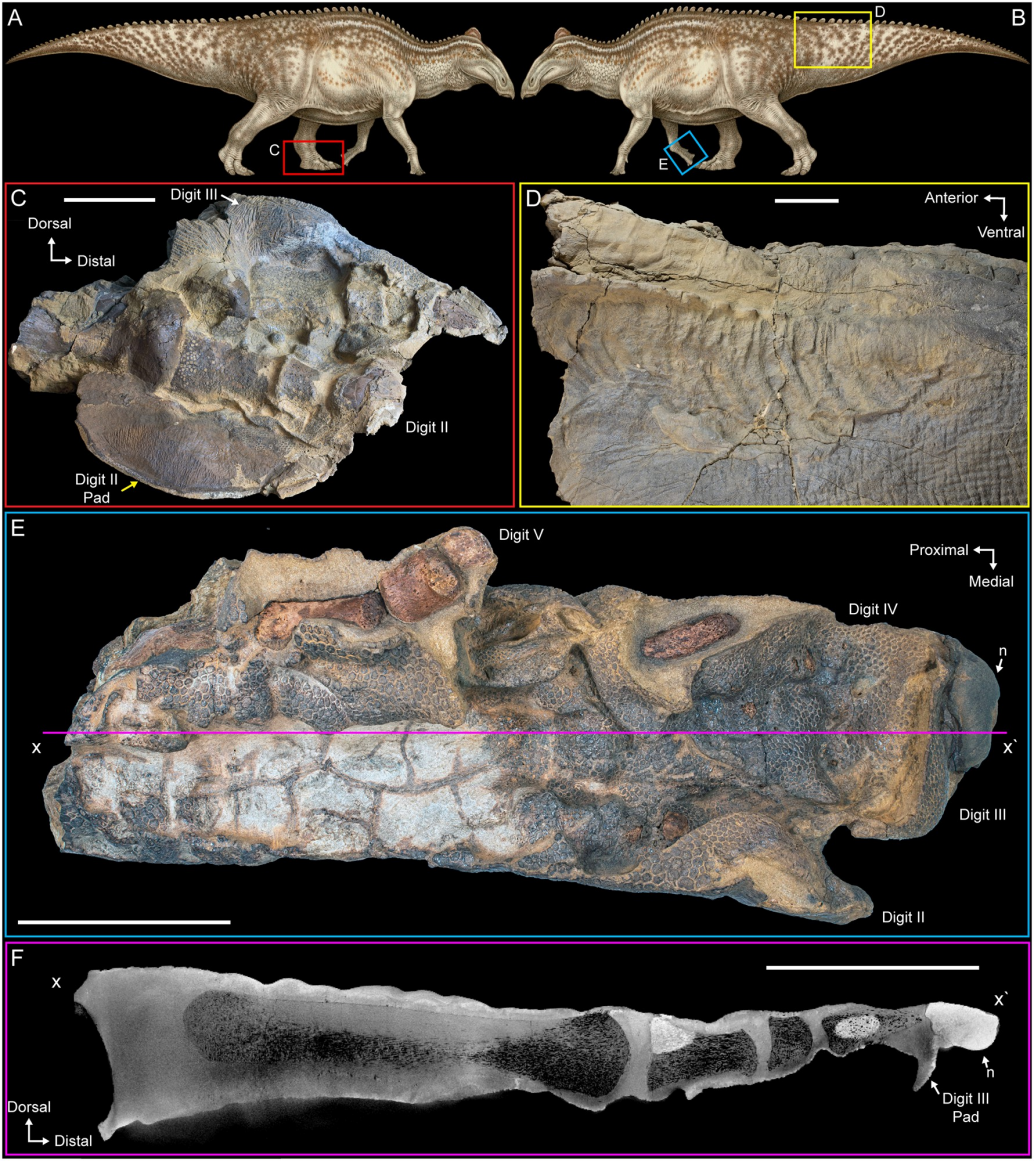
Evidence of desiccation in NDGS 2000. (A) NDGS 2000 reconstruction in right lateral view. (B) NDGS 2000 reconstruction in left lateral view. (C) Left pes in medial view. (D) left lateral view of the proximal portion of the tail. (E) Right manus in ventral view. (F) CT image of cross section of right manus along line x to x’ in (E). Colored boxes and lines in (A), (B), and (E) indicate the locations and views of the photographs in the other figure parts. Paleoart in (A) and (B) by Natee Puttapipat. All scale bars equal 10 cm. Abbreviations: n, iron oxide nodule.
